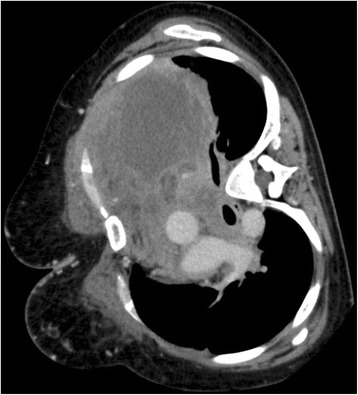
Computed tomography scan below the level of carina, which was taken on day 2 after admission, showing severe obstruction of the left main bronchus
